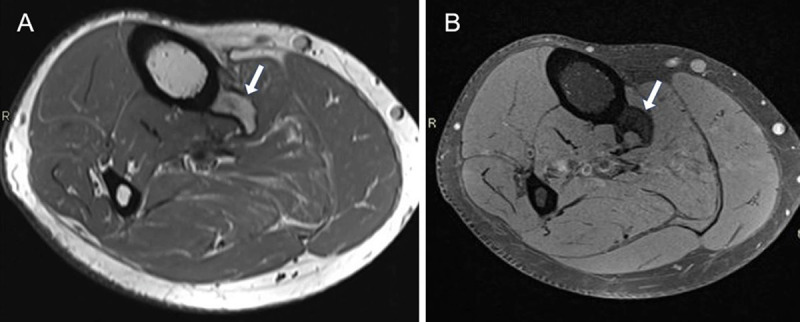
Subsequent CT examination confirmed the presence of a bony excrescence at the posteromedial tibial diaphysis with bifid morphology (*Figure 2A*, axial). A 3D reconstruction showed that the lesion was predominantly located on the course of the attachment of the soleus muscle at the tibia with an additional smaller component at the fibular attachment of the soleus (*Figure 2B*, posterior view, arrows). The diagnosis of a giant tug lesion of the soleus was made.
